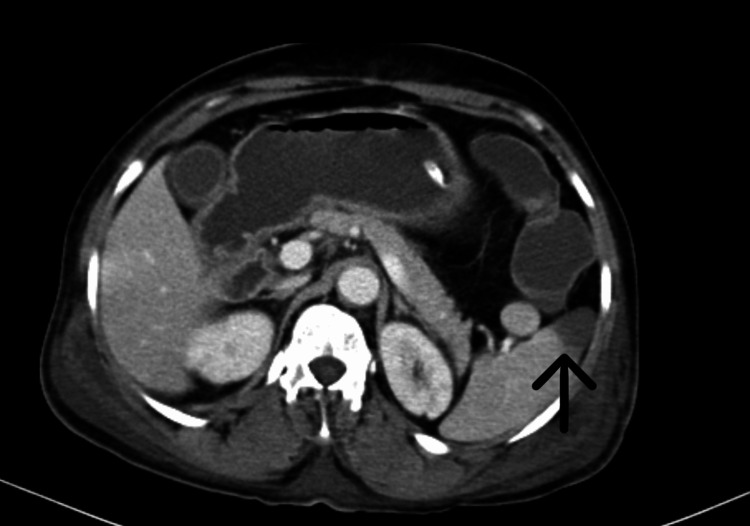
Contrast-enhanced computed tomography (CECT) of the abdomen (axial view) The black arrow shows splenic infarct
